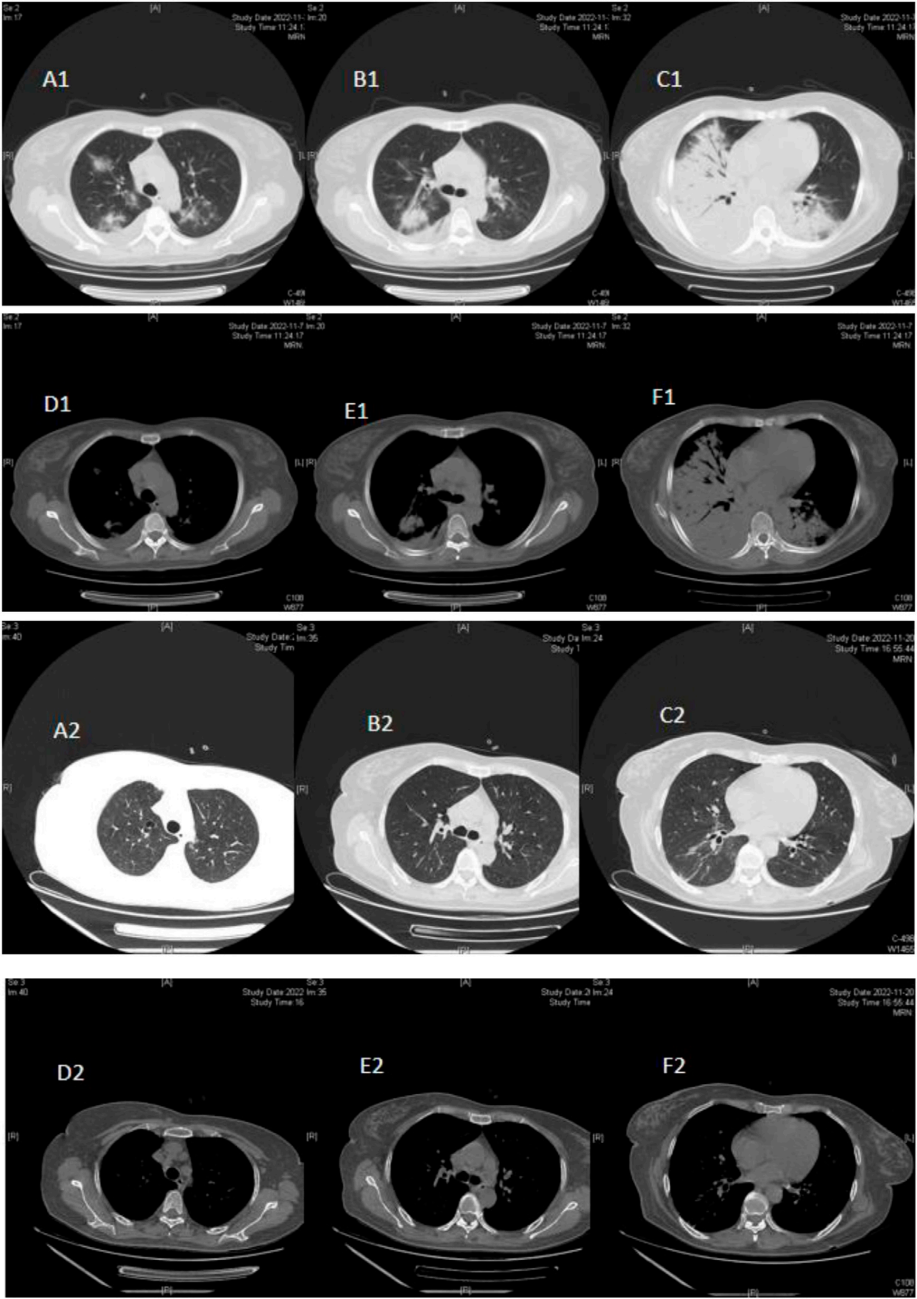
Case 3, a 50-year-old female, was hospitalized on 7 November, 2022, due to a “cough and fever for 6 days.” On admission (November 7), pulmonary CT showed, double lower lung consolidation, double upper lung ground-glass change, consolidation (mainly right lung lesions), and a small amount of pleural effusion (A1–F1) on the right side. After doxycycline treatment, the lung CT reexamination on 20 November, 2022, showed that the bilateral lung lesions were absorbed and improved (A2–F2).
